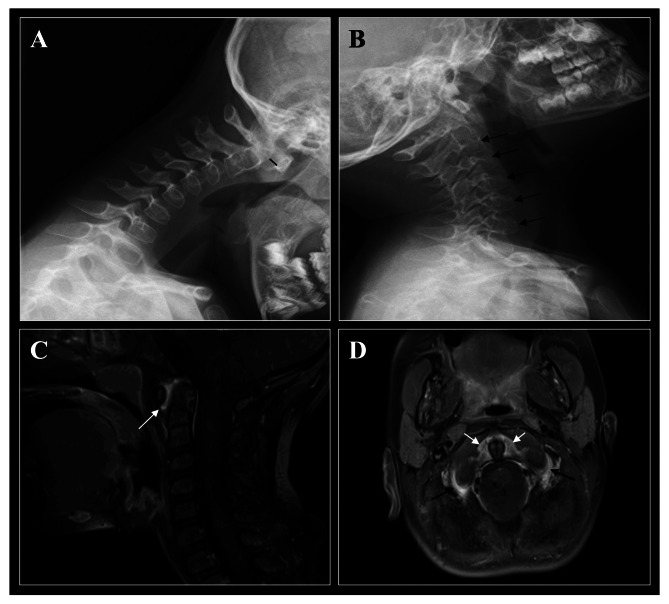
Representative images of the cervical spine involvement in a 4-year-old patient with oligoarticular JIA. (A/B) Lateral-view radiographs of the cervical spine at diagnosis (flexion/extension). (A) Cervical spine in flexion shows anterior atlantoaxial subluxation of 5.6 mm (black line). (B) Cervical spine in extension shows diffuse demineralization at C2-C6 vertebral bodies (black arrows). (C/D) MRI of the cervical spine of the same patient at diagnosis, both images of T1 sequences with fat saturation and with a contrast medium. (C) Sagittal section of the cervical spine shows an intense contrast enhancement and synovial hypertrophy in the atlanto-axial joint (white arrow). (D) Axial section of the cervical spine shows an intense contrast enhancement and synovial hypertrophy in the atlanto-axial joint in the pre- and paradental space (white arrows) and contrast enhancement in the lateral masses (both right and left) of the atlanto-axial joint (black arrows)
